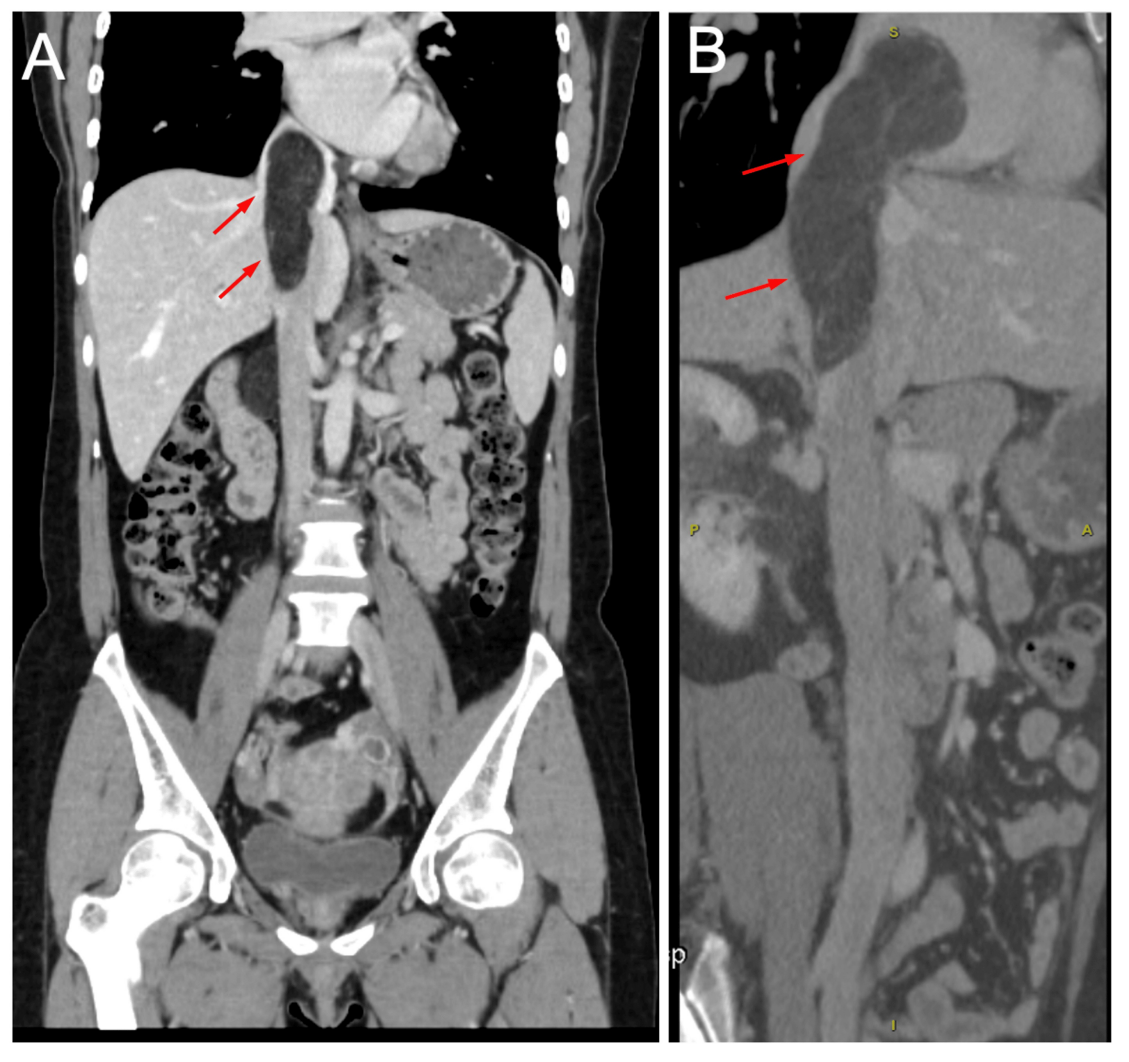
Coronal and two-dimensional reconstruction views of the chest and abdominal computed tomography (CT) scan of the patient on admission. (A) Coronal view of CT scan showing a hypodense tumor within the inferior vena cava (IVC). (B) Two-dimensional reconstruction view of the CT scan showing the hypodense tumor in IVC with right cardiac extension.
